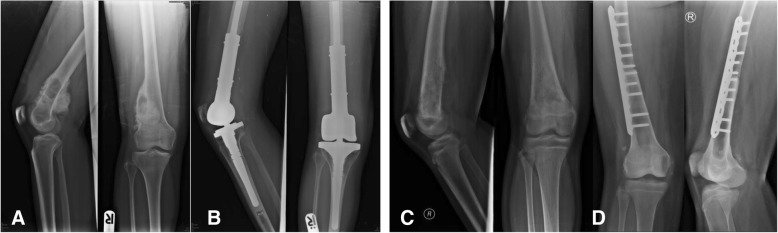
Two comparison distal femoral osteosarcoma cases treated without using 3D model for assistance. a The first case showing the x-ray finding of the dedifferentiated parosteal osteosarcoma right distal femur, (b) Performed wide resection of 17.5 cm length and reconstructed by rotating hinge knee endoprosthesis, (c) The second case showing the x-ray finding of conventional osteosarcoma right distal femur, (d) Performed wide resection of 20 cm and reconstructed with the distal femoral allograft and locking compression plate
